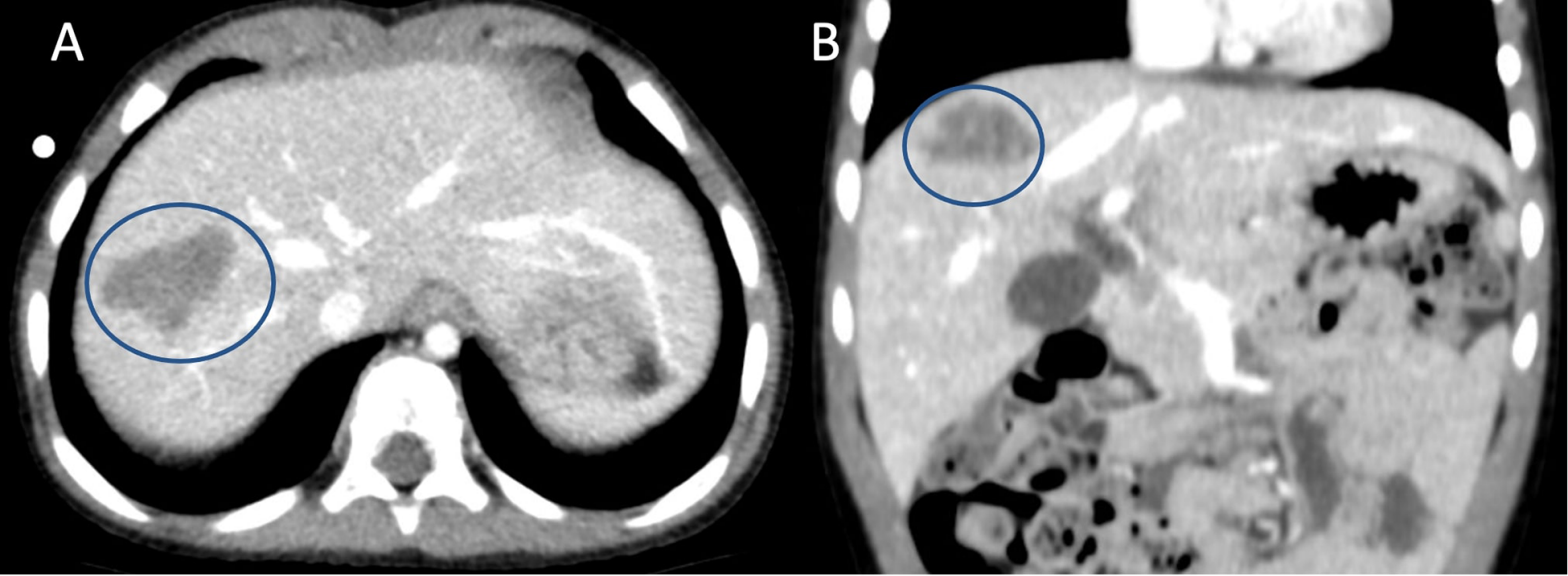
Preoperative CT-scan showing the hepatoblastoma (encircled by the blue line) located in segment 7 near the right hepatic vein. (A) axial view, (B) coronal view.
